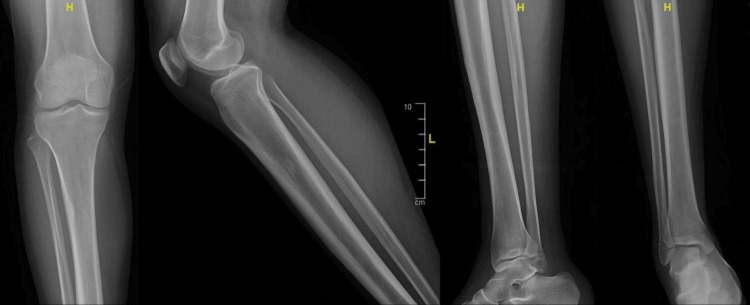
Radiograph showing no focal bony or joint abnormalities and no evidence of a fracture
